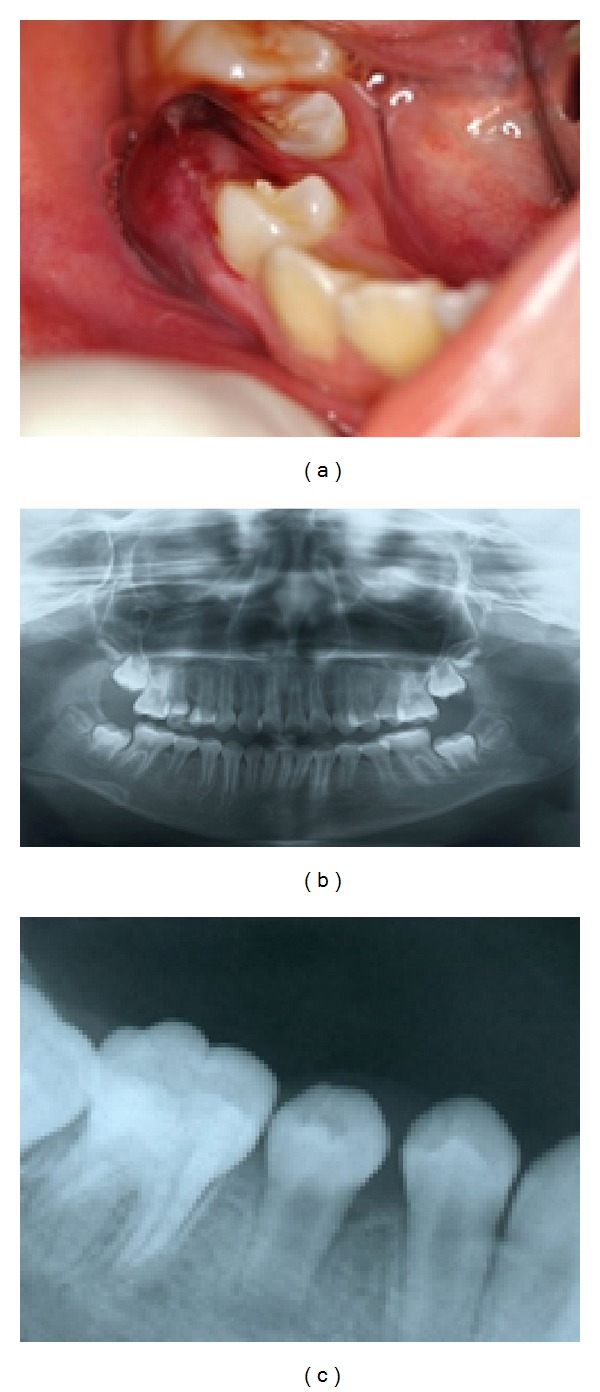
(a) Preoperative intraoral photograph showing a gingival abscess in the mandibular right second premolar. (b) Panoramic X-ray showing extensive radiolucency in the periradicular region in the mandibular right second premolar compared with the mandibular left second premolar. (c) X-ray showing an immature open apex and enlargement of the periodontal ligament space and extensive radiolucency in the periradicular region in the mandibular right second premolar.
